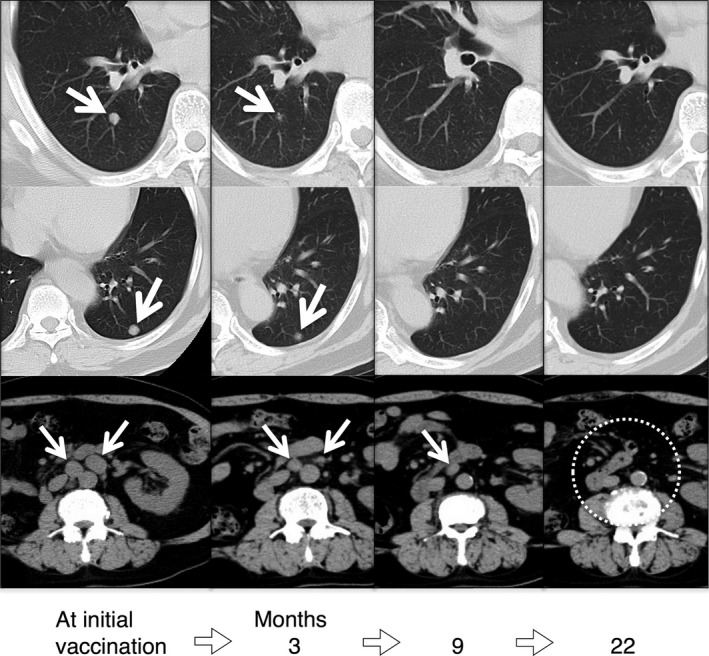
CT images in the metastasized lung and para‐aortic lymph node lesions. Multiple metastases to lung and para‐aortic lymph nodes were detected at the initial vaccination with AFTV. Three months after the first vaccination with AFTV, all of the metastatic lesions showed a tendency to decrease in size. Nine months after the first vaccination with AFTV, disappearance of the metastases in lung and further reduction of para‐aortic lymph node metastases were detected. Twenty‐two months later, all metastases disappeared from the image.
